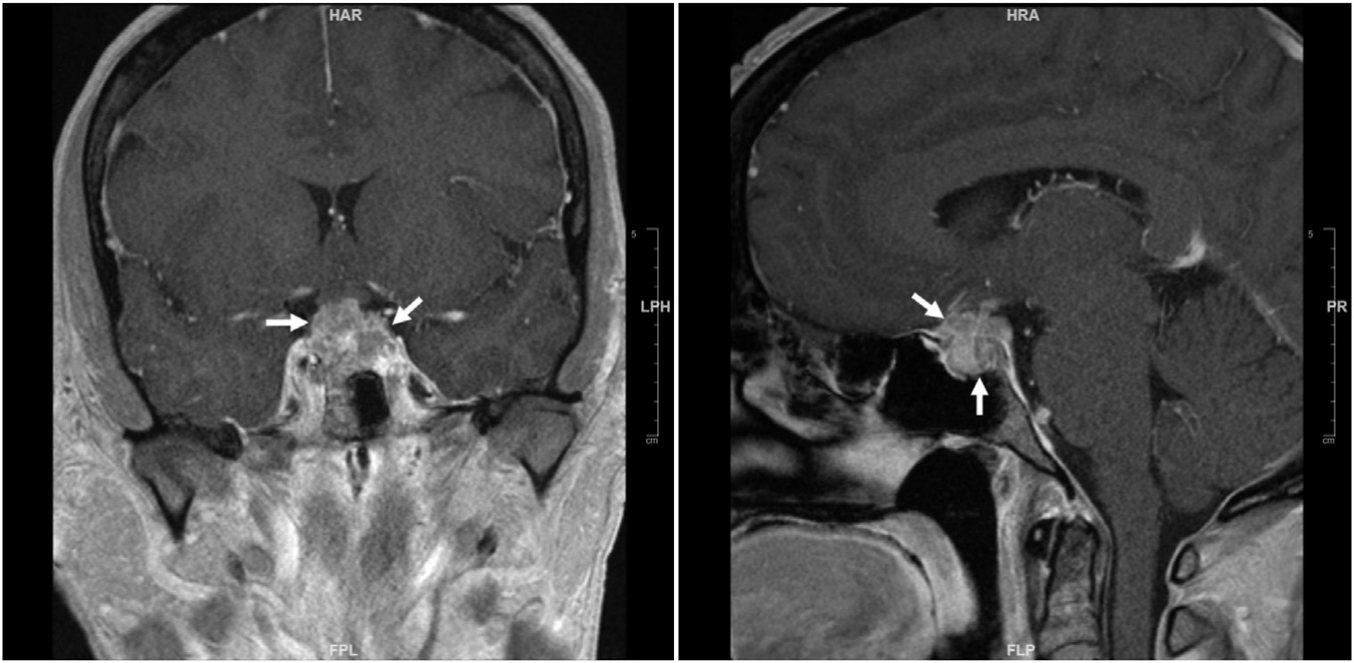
Pituitary magnetic resonance imaging of patient 1 at initial presentation. Left, coronal view; right, sagittal view. The tumor invaded into the right cavernous sinus, circumferentially encased the cavernous right internal carotid artery, abutted the left cavernous sinus and the left internal carotid artery, and slightly displaced the optic chiasm. Arrows indicate the tumor.
